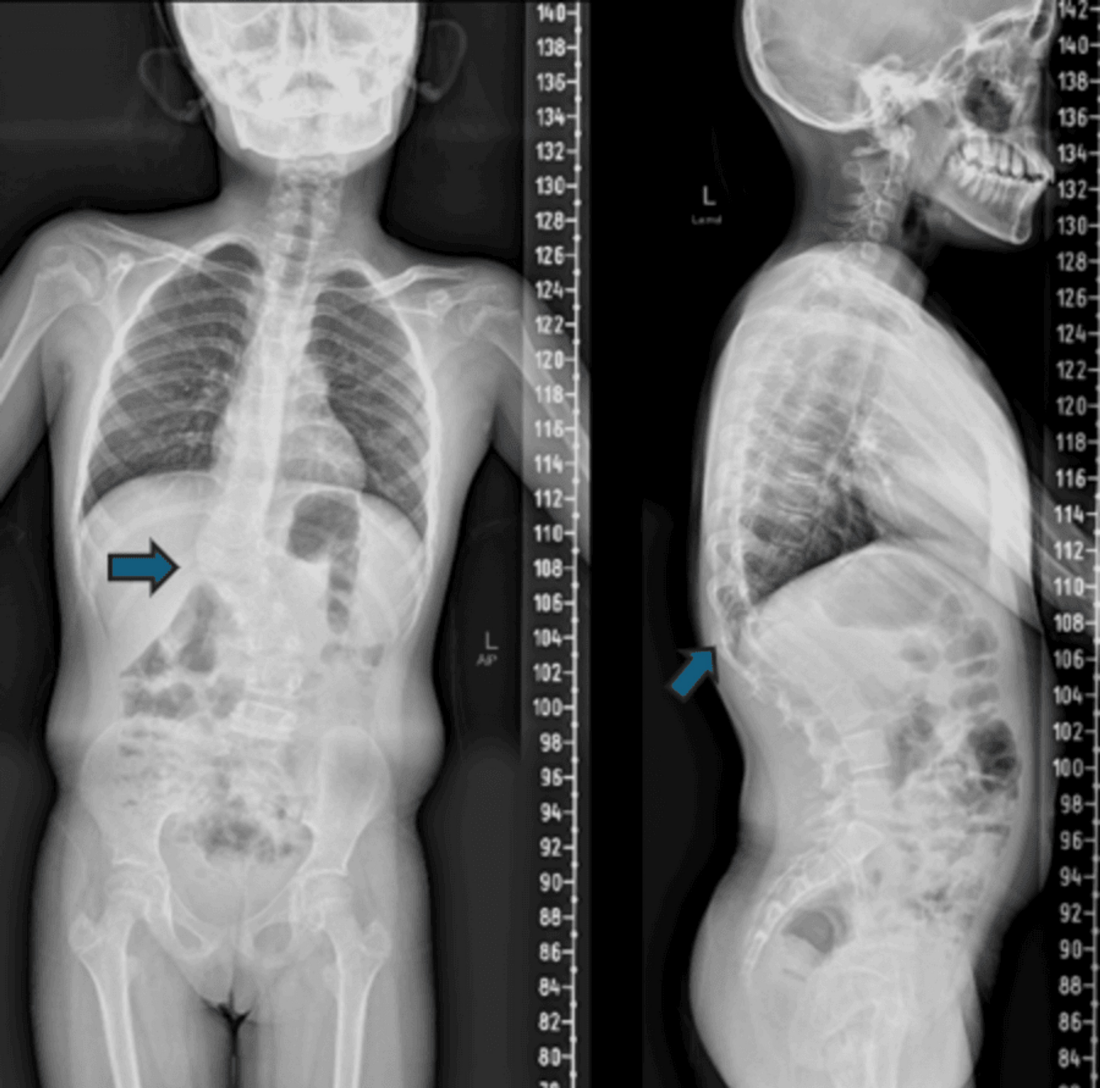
Anteroposterior and lateral views of the whole spine. X-ray shows thoracolumbar scoliosis with kyphotic deformity primarily at the T12 vertebra. Cobb’s angle of 43 degrees from T10 to T12 and Cobb’s angle of 41 degrees from L1 to L4 can be seen. The kyphosis angle of 52.5 degrees can be noted from T11 to L1.
